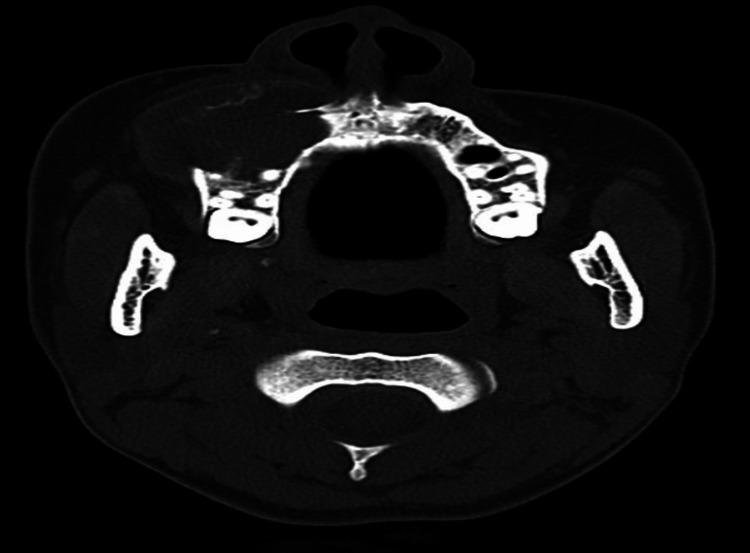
Axial section CT demonstrating a well-defined mixed radiolucent-radiopaque expansile lesion of size 3.5 × 3.4 × 3.8 cm (AP × ML × SI), destruction of labial, buccal cortical plate, and thinning of palatal cortical plate CT: computed tomography, AP: anteroposterior, ML: mediolateral, SI: superoinferior
